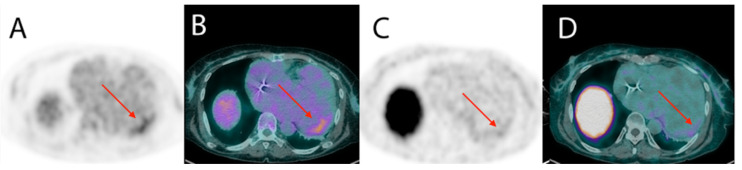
Increased cardiac volume in a patient with Chagasic cardiomyopathy. Mild increase in uptake of 18F-FDG (A,B) and of 68Ga-DOTATOC (C,D) in positron emission tomography (PET)-CT images was restricted to the basal anterolateral segment, indicating the presence of inflammation in this segment.
